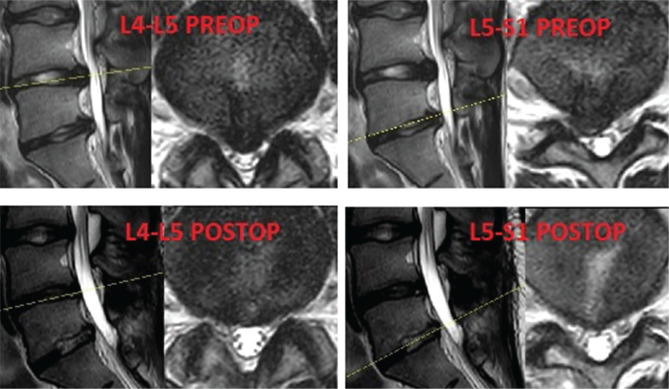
Preoperative and postoperative magnetic resonance images.
